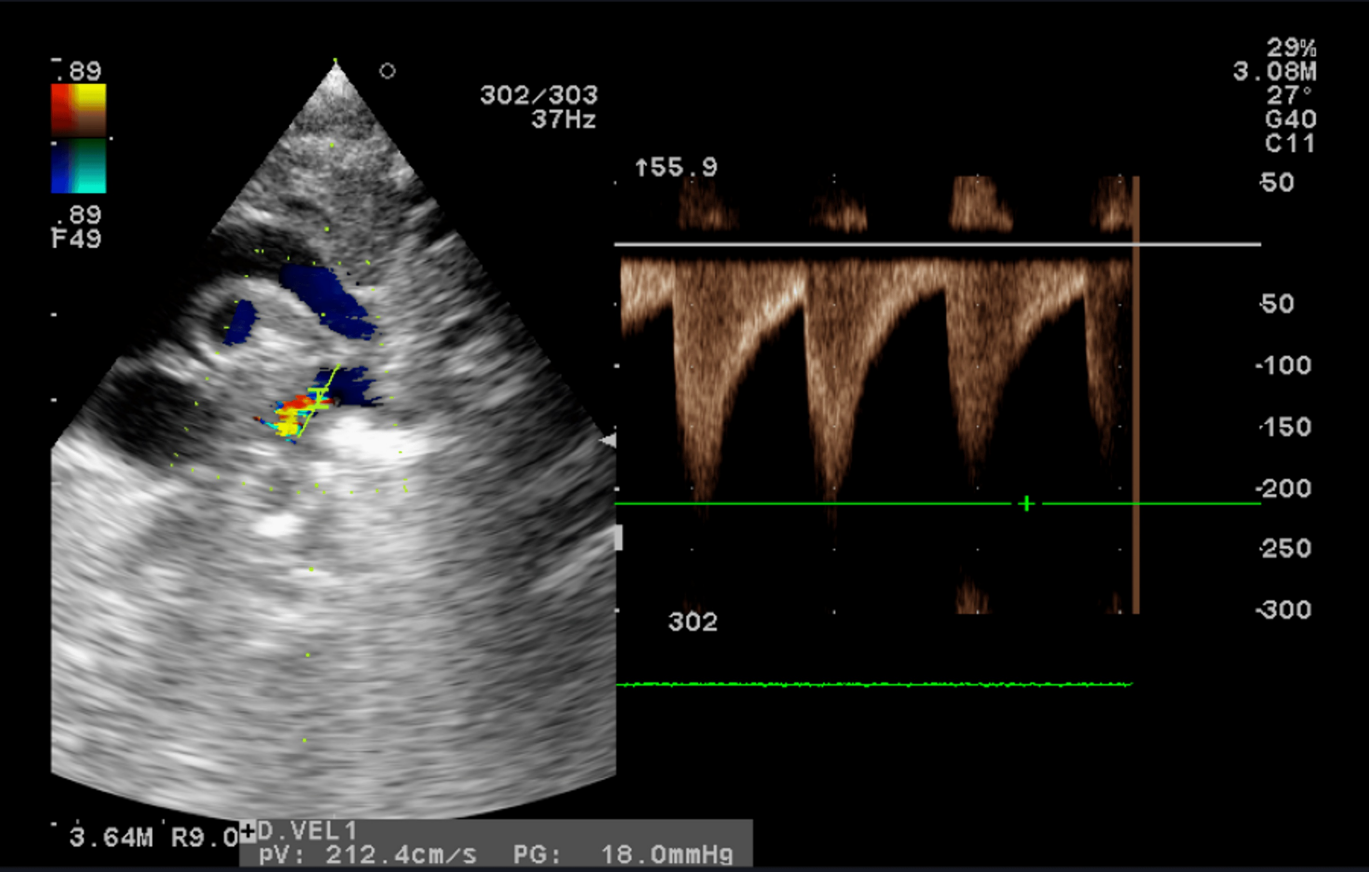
Echocardiography (Eco 2D/ Doppler) on day 5 of life (Case 1): CoA flow on aortic isthmus CoA: coarctation of the aorta
